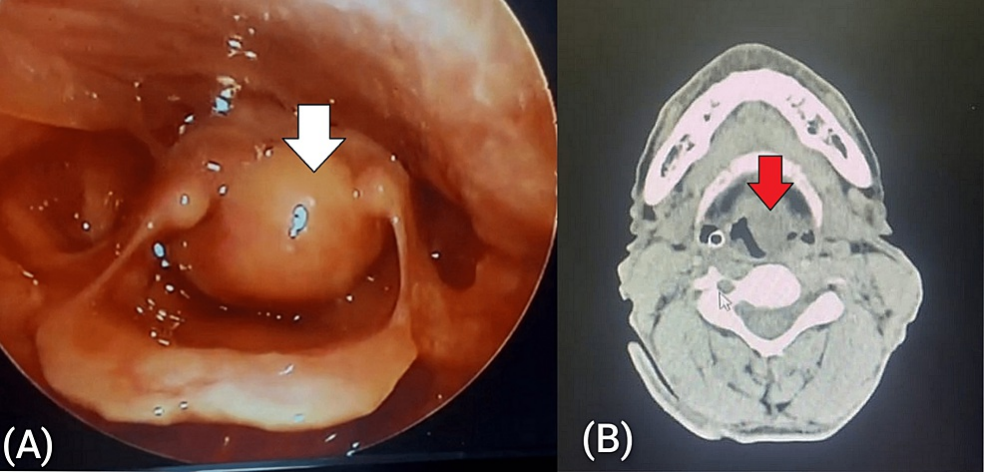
(A) Endoscopic image of laryngeal pleomorphic adenoma involving false cord; (B) CT image (axial section) of laryngeal pleomorphic adenoma CT: computed tomography
